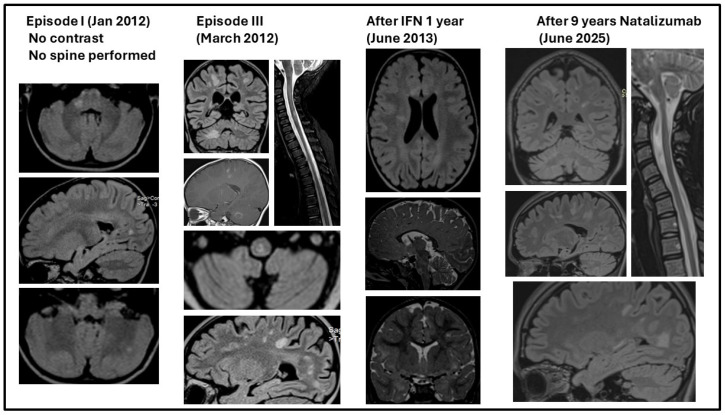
MRI at onset and in evolution.
